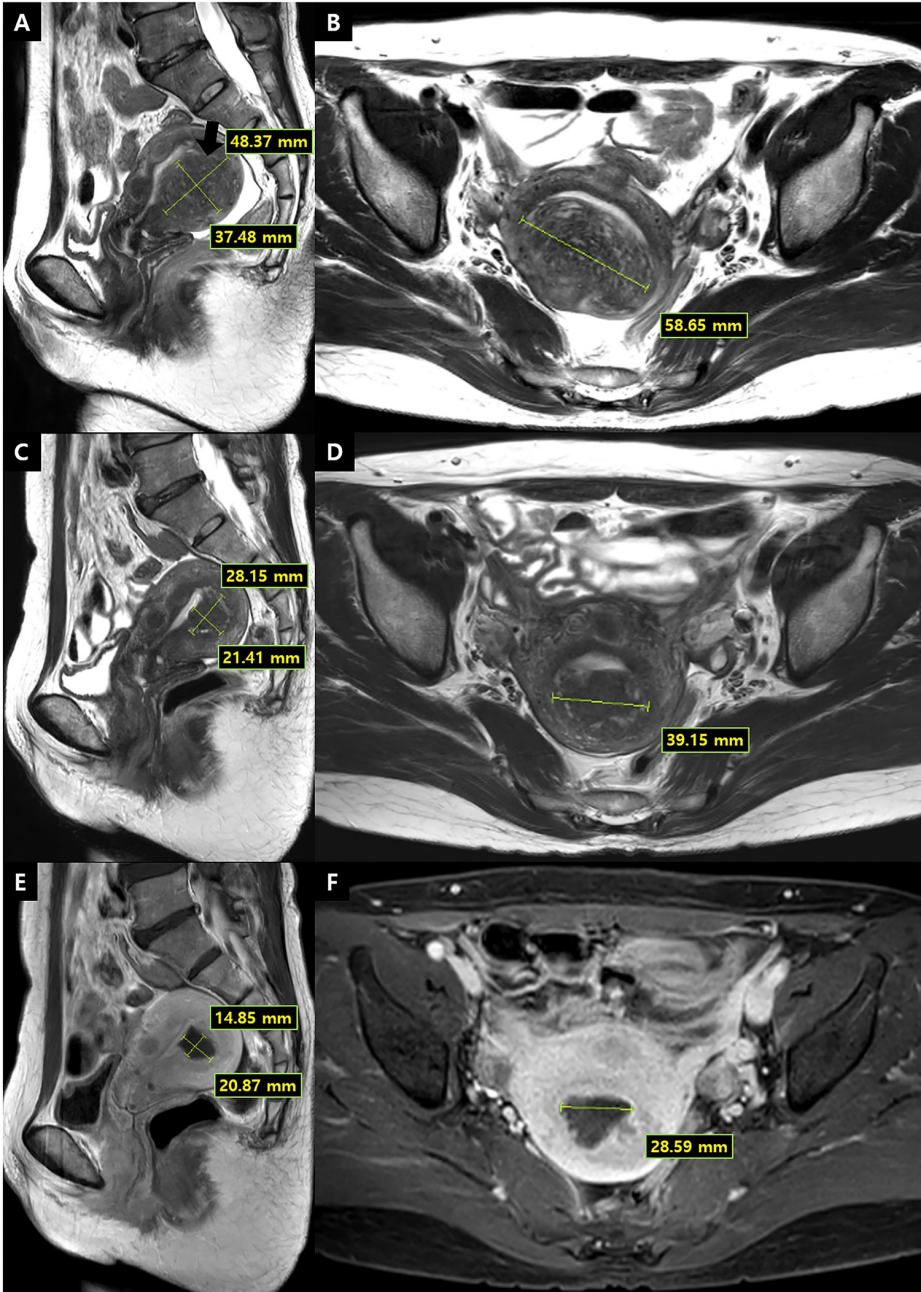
Examples of quantitative assessment of treatment efficacy on MR images of a 50-year-old woman. (A, B) Pre-HIFU sagittal and axial T2-weighted images show an adenomyotic lesion at the posterior wall of the uterus. The volume of the adenomyotic lesion was calculated as 0.523 × length × width × height (lines) and was equal to 60.8 cm^3^. (C–F) Three-month follow-up images of the same participant. (C, D) Sagittal and axial T2-weighted images show shrinkage of the adenomyotic lesion. The adenomyosis volume was calculated as 12.0 cm^3^, and the adenomyosis volume shrinkage rate was 80.3%. (E, F) Contrast-enhanced T1-weighted images demonstrate nonperfused volume, which was equal to 8.3 cm^3^. The calculated nonperfused volume ratio in this participant was 69.1%.
